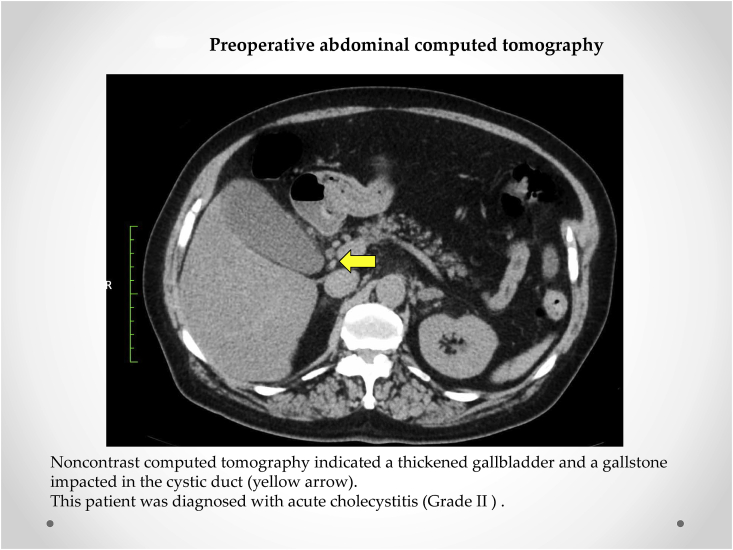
Preoperative abdominal computed tomography. Non-contrast computed tomography indicates a thickened gallbladder and a gallstone impacted in the cystic duct (yellow arrow). This patient was diagnosed with acute cholecystitis (Grade II). . (For interpretation of the references to colour in this figure legend, the reader is referred to the Web version of this article.)
